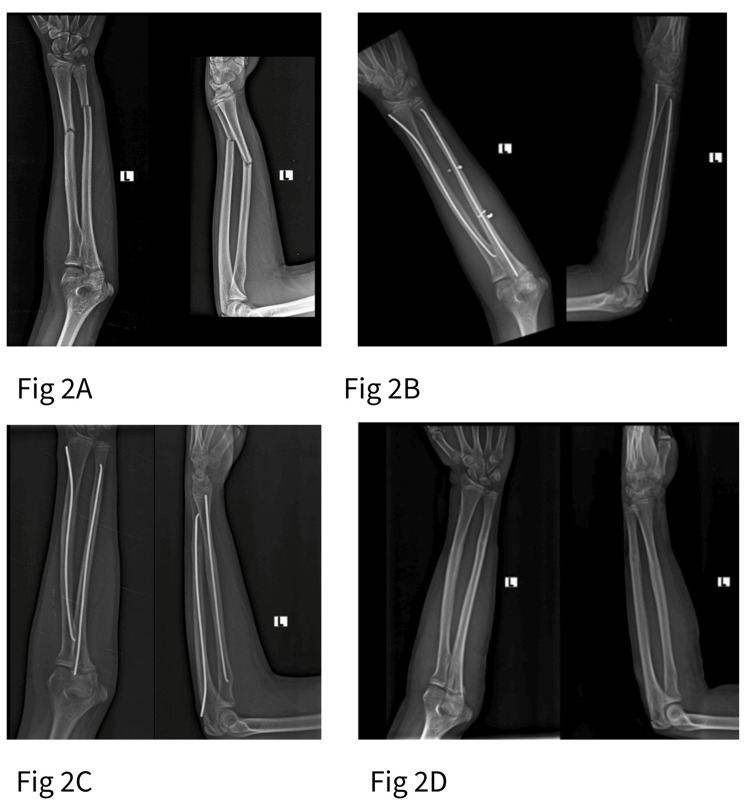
Plain radiographs representing antegrade titanium elastic nailing for left ulna fracture A: Pre-operative; B: Immediate post-operative; C: 6 months follow-up with complete union; D: post implant removal.
